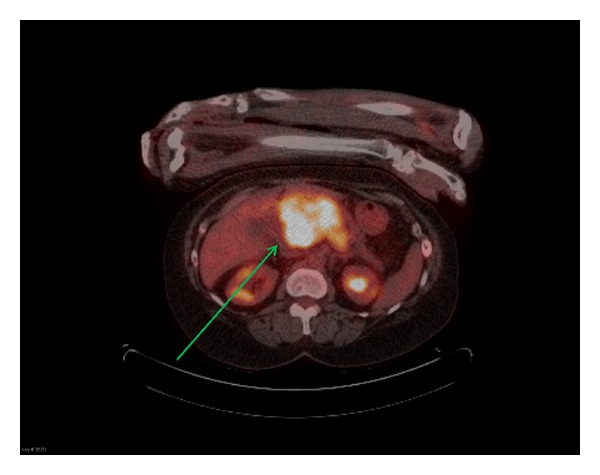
Axial PET/CT fusion image demonstrates a large, intensely FDG avid mass in the region of the pancreas (green arrow).
